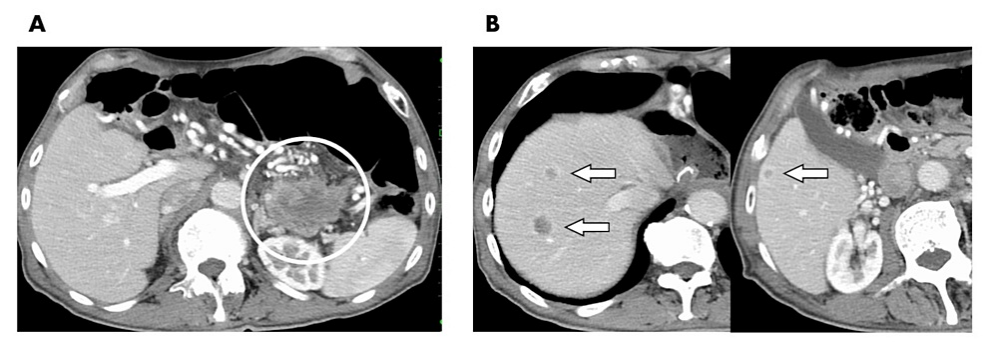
Pretreatment computed tomography images. (A) Pancreatic tail tumor (circle) and (B) metastases in liver segments 8 and 6 (arrows).
